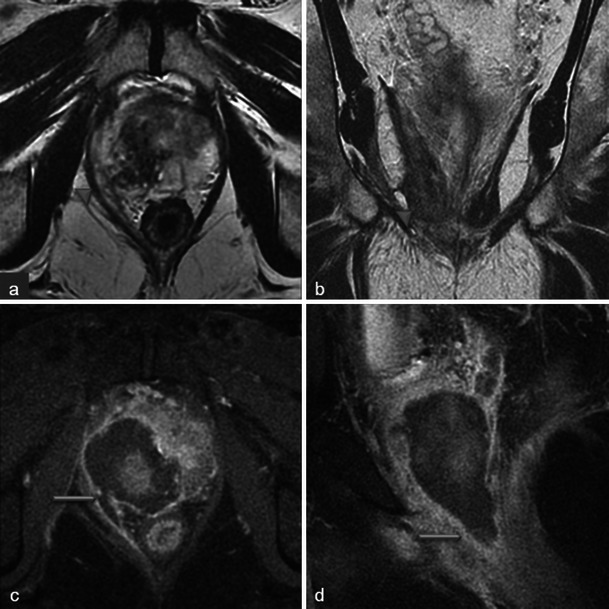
Levator ani muscle. Right photodynamic therapy in a 65-year-old patient for Gleason 6 adenocarcinoma (two positive biopsies in right prostate). Day-7 post-treatment axial (a) and coronal (b) T2-weighted MR images visualise a heterogeneous high signal of the right puborectalis muscle (*arrowheads*); axial and sagittal T1 FS contrast-enhanced images (c, d) show a low-signal well-defined area surrounded by a hyperintense enhanced border after gadolinium injection (*arrows*) corresponding to extraprostatic necrosis affecting the right levator ani muscle
